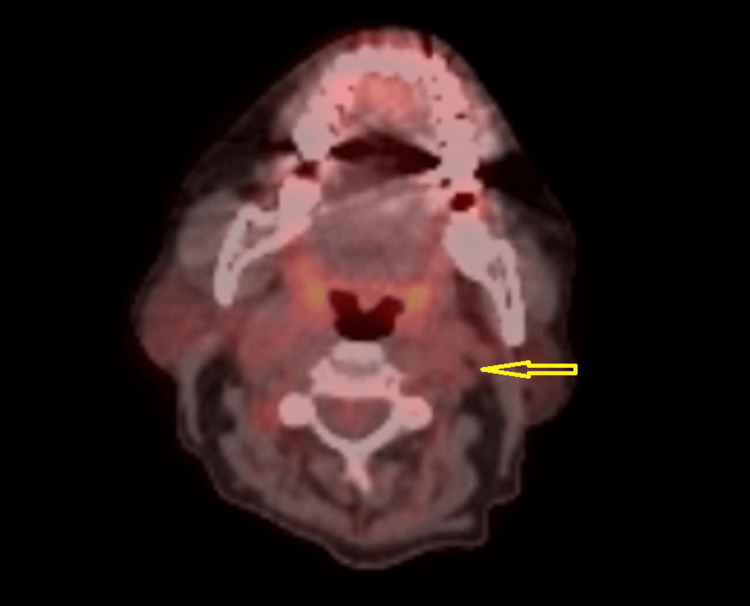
Fluorodeoxyglucose positron emission tomography (FDG PET) scan after treatment Fused axial PET view, performed eight weeks after the first cycle, showing complete resolution of left cervical lymphadenopathy following two cycles of chemotherapy (arrow).
